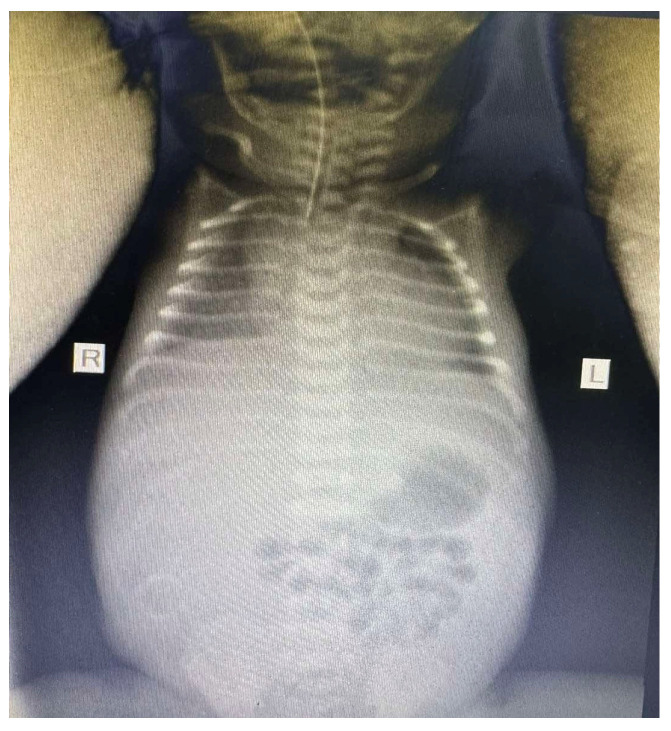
Chest and abdominal X-ray demonstrating neonatal respiratory distress syndrome (NRDS) and hepatosplenomegaly.
